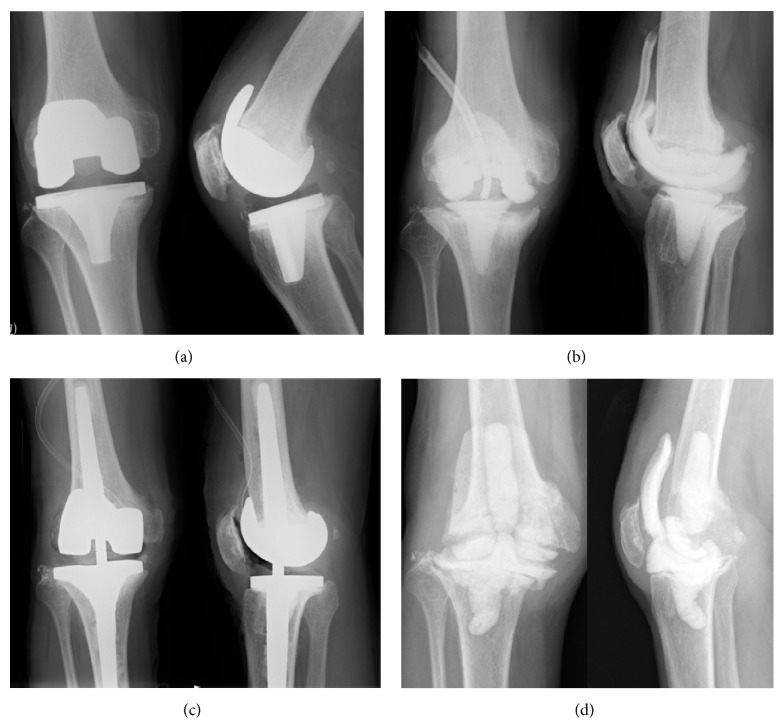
(a) Anteroposterior radiograph of right knee after primary TKA. (b) Anteroposterior radiograph of right knee after first antibiotic-impregnated cement spacer exchange and debridement. (c) Anteroposterior radiograph of right knee after revision TKA. (d) Anteroposterior radiograph of right knee after second antibiotic-impregnated cement spacer exchange and debridement.
